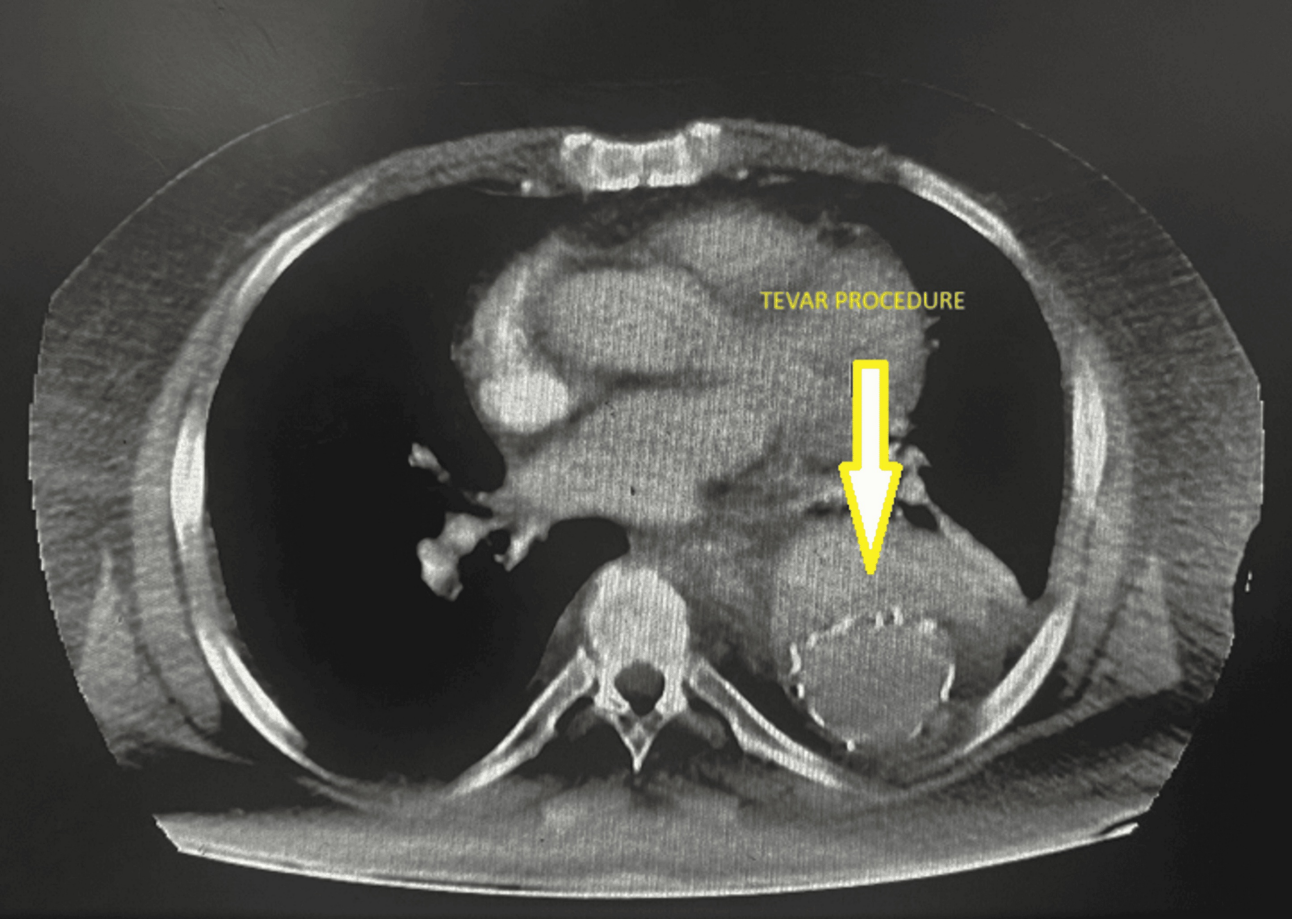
Post-TEVAR This image shows a CT scan of the thorax (axial view). The yellow dart points to a region labeled TEVAR, which indicates thoracic endovascular aortic repair. CT: computed tomography, TEVAR: thoracic endovascular aortic repair
